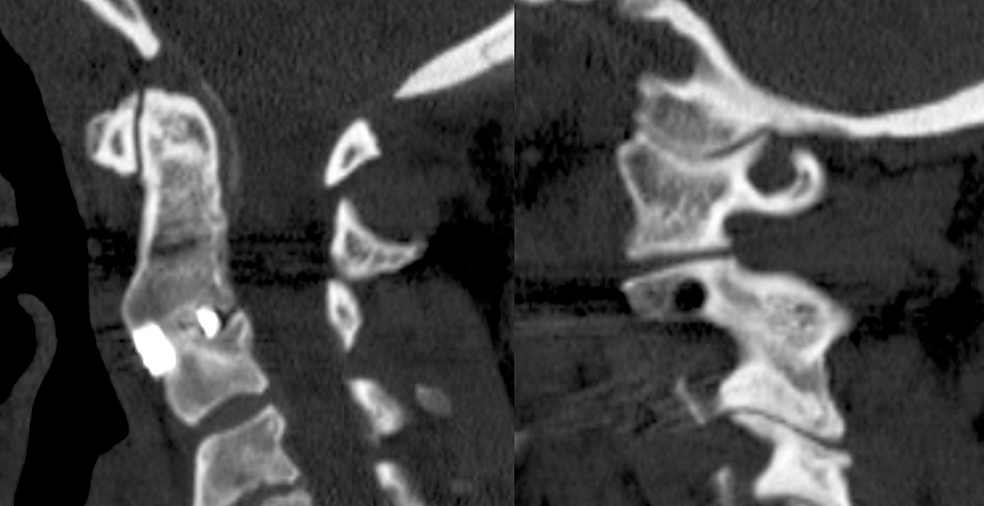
A CT scan midline sagittal reconstructed image at one-year post-surgery showing a solid arthrodesis across the C2/3 disc space (left). Interestingly, a parasagittal image shows that the fracture line has also healed and the facet joint has fused, presumably because of the immobilization of the spinal segment resulting from the anterior fusion. CT: computed tomography
